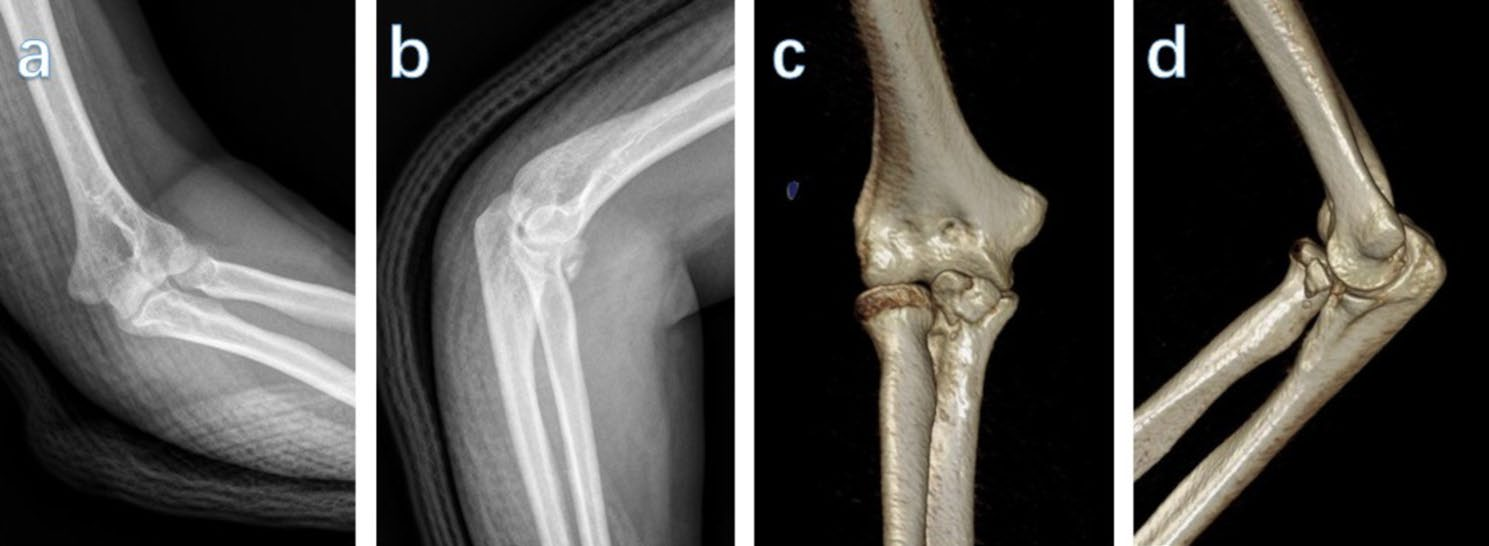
Representative Regan-Morrey type III coronoid fracture. (a, b) Preoperative radiographs of the coronoid process fracture. (c, d) Reconstructed three-dimensional computed tomography images of the coronoid process fracture
